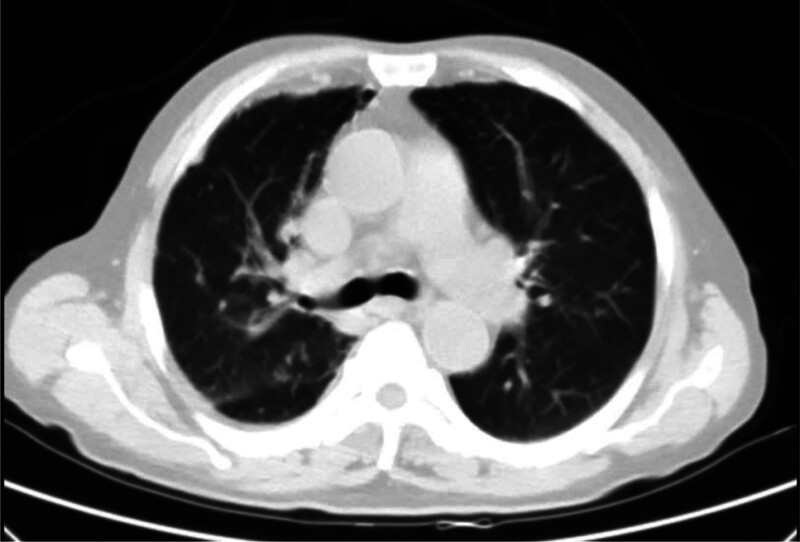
Chest CT showed interstitial changes in both lungs with chronic inflammation. CT = computed tomography.
